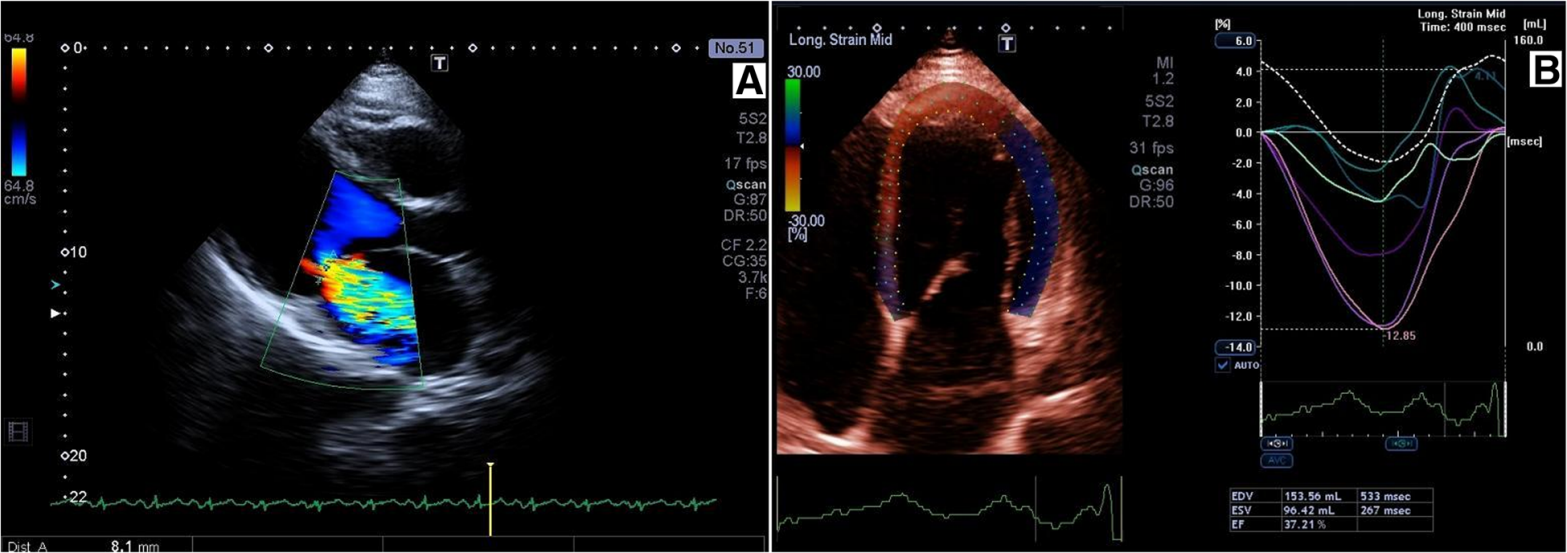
Two-dimensional echocardiographic study of proband III-1. A: Parasternal long axis view in systole with color flow Doppler. Severe mitral valve regurgitation (vena contracta of 8 mm) due to restriction of the mitral valve leaflets. B: Apical four-chamber view, speckle tracking method. Enlarged left ventricle, left ventricular end-diastolic volume (LVEDV 154 ml) with low ejection fraction (LVEF 37%). Enlarged left atrium chamber.
